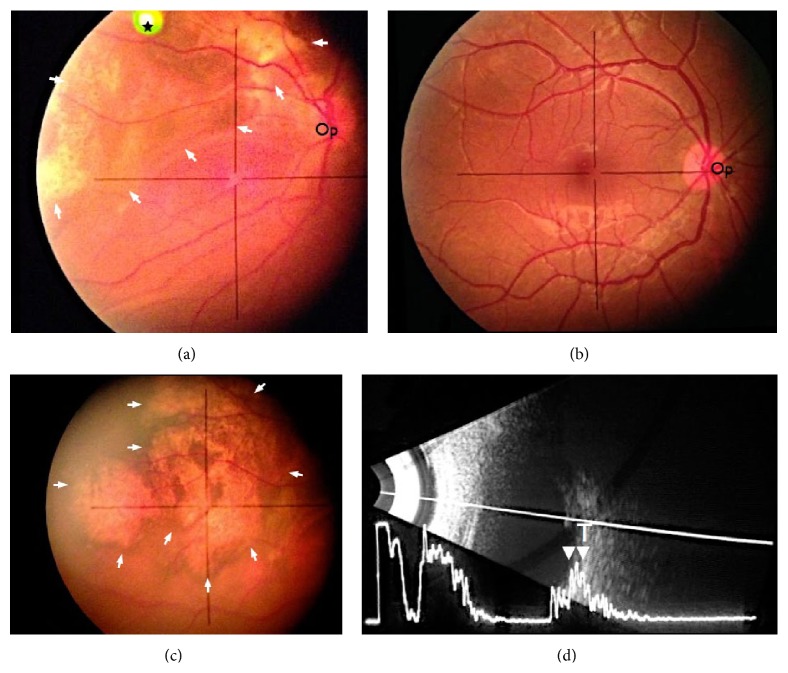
Selected fundoscopy images of the retina (a) at the level of the left optic nerve (Op) and (b) at the level of the right optic nerve (Op); (c) left nasal equatorial retina; (d) ultrasound B-scan with vector A scan. Images (a) and (c) show multiple ill-defined tumors with irregular surface (marked with white arrows in images (a) and (c)). Image (b) shows the comparative normal appearances of the right side. Image (d) shows the retinal lesion (T) with high intralesional reflectivity (white arrowheads). ★ is artifact.
